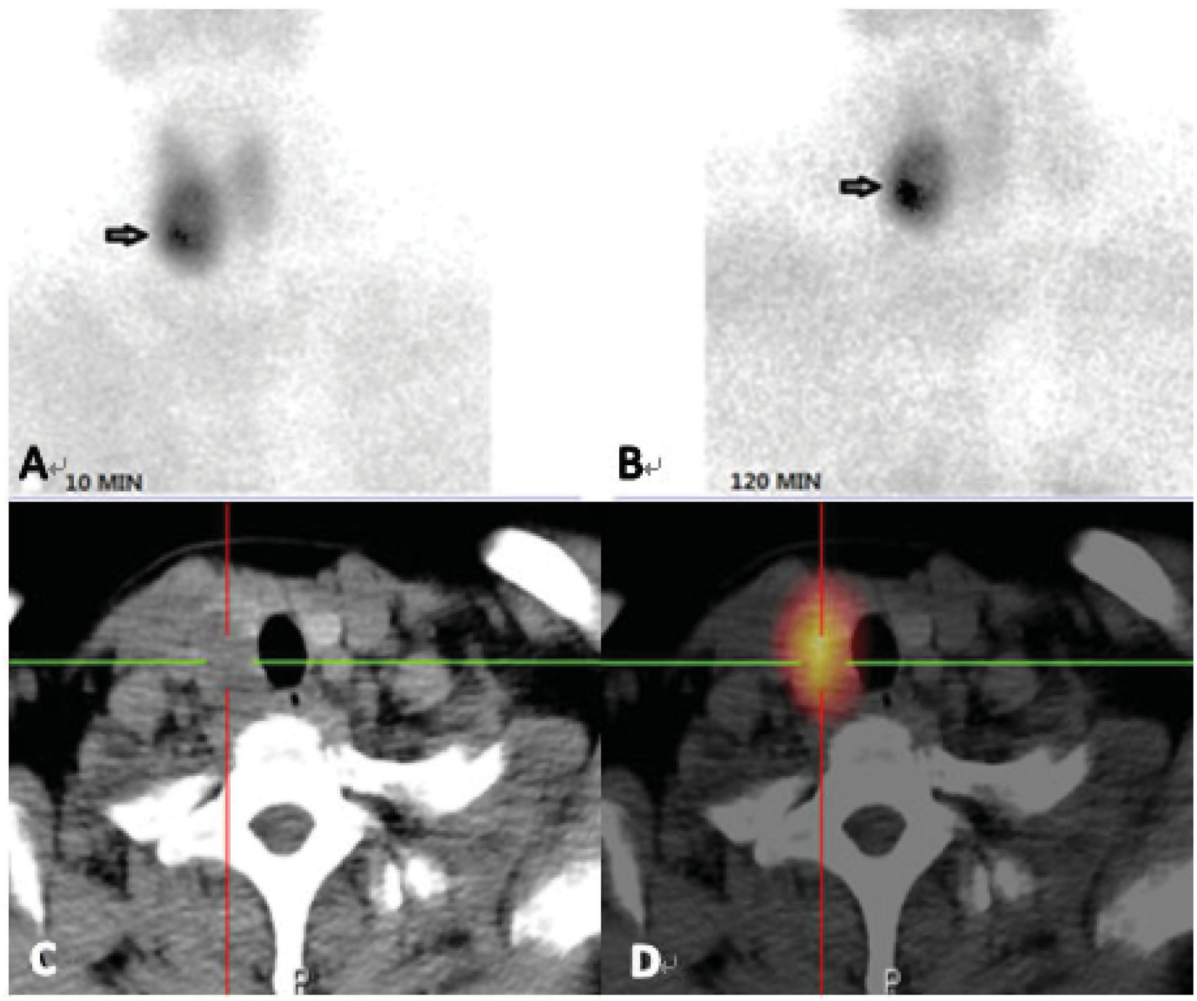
Images from a 41-year-old female diagnosed with PHPT. Her serum levels of iPTH and calcium on admission were 726.80 pg/mL and 2.97 mmol/L, respectively. (A, B): Dual phase ^99m^Tc-MIBI planar scintigraphy of the parathyroid glands, (A) 10min, (B) 120 min. CT alone (C) and SPECT/CT (D) of the same cross-section, showing the right inferior parathyroid. The best diagnostic performance is achieved by combination of a detailed morphological image (CT) and functional, scintigraphic map (SPECT/CT).
